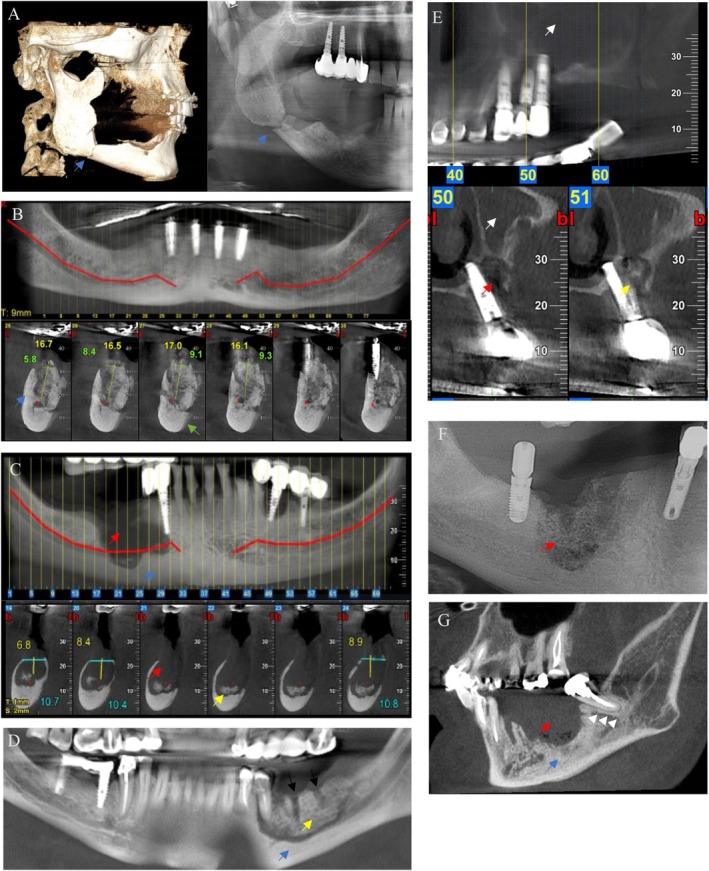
Representative radiographic features of MRONJ. (A) Right mandibular pathological fracture (blue arrow); left: Three‐dimensional MDCT reconstruction, right: Panoramic radiograph. (B) Right mandibular sclerosis (blue arrow), lytic lesion (red arrow), and subperiosteal reaction (green arrow); upper: Panoramic CBCT reconstruction, lower: Axial CBCT sections. (C) Extensive right mandibular lysis (red arrow), sclerosis (blue arrow), and sequestration (yellow arrow); upper: Panoramic CBCT reconstruction, lower: Axial CBCT sections. (D) Left mandibular sequestration (yellow arrow), sclerosis (blue arrow), and unhealed extraction sites (black arrows); panoramic CBCT reconstruction. (E) Left maxillary sequestration (yellow arrow), sclerosis (red arrow), and maxillary sinus opacification (white arrow); upper: Panoramic CBCT reconstruction, lower: Axial CBCT sections. (F) Extensive right mandibular lysis (red arrow); panoramic CBCT reconstruction. (G) Extensive left mandibular lysis (red arrow), sclerosis (blue arrow) and thickening of the lamina dura (white arrow heads); MDCT.
